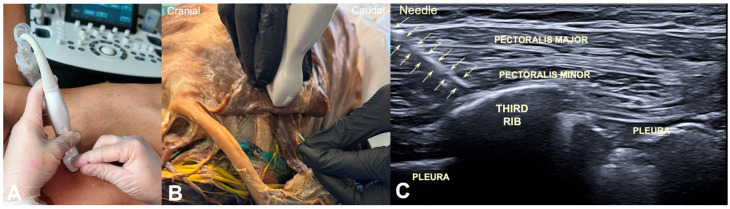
(A) Ultrasound-guided in-plane (long-axis) flat-needle approach in a human subject. (B) Ultrasound-guided in-plane (long-axis) flat-needle approach in the cadaver showing the pectoralis minor for confirmation. (C) Longitudinal-axis ultrasound image showing the needle (arrows) in the muscle belly of the pectoralis minor muscle with the tip towards the third rib. PM: pectoralis major muscle; Pm: pectoralis minor muscle.
